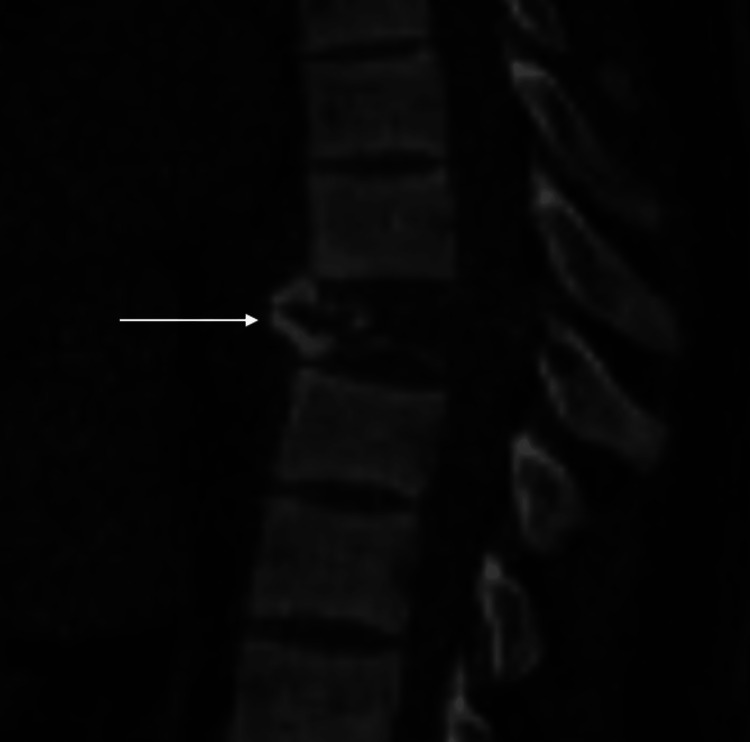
Computed tomography (CT) of the spine sagittal, T8 vertebral body expansile lytic lesion with collapse and impending kyphosis, as indicated by the arrow
